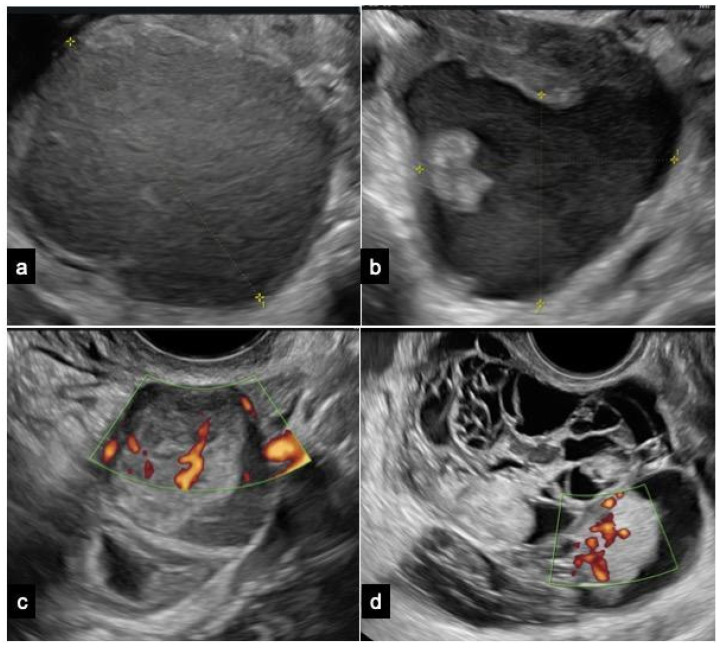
Ultrasound images of (a) a typical endometrioma, (b) an atypical endometrioma, (c) a clear cell carcinoma, and (d) an endometrioid carcinoma. The ultrasonographic diagnosis was confirmed by pathological examination, and patients provided informed consent for the use of their images.
